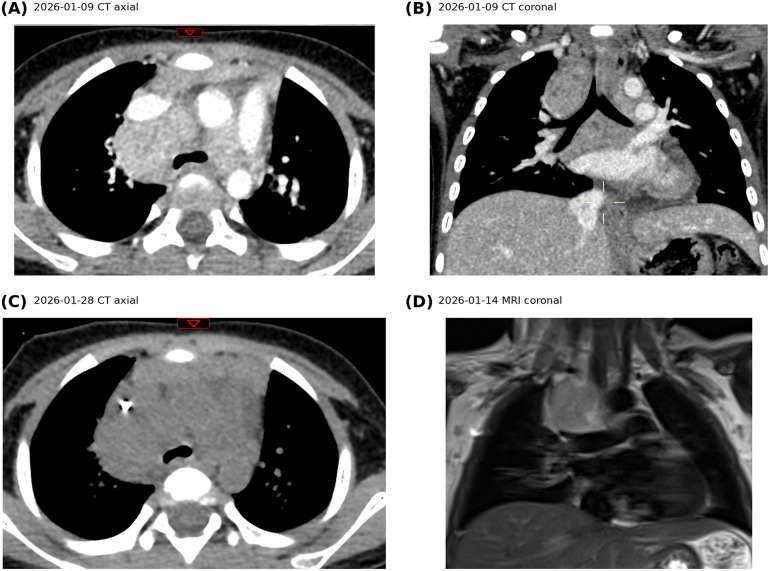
Representative imaging findings during hospitalization. (A) Axial contrast-enhanced chest CT on January 9, 2026 showing a mediastinal–hilar mass-like lesion with heterogeneous enhancement and airway compression. (B) Coronal contrast-enhanced CT on January 9, 2026 showing the relationship of the lesion to the airway, hila, and adjacent great vessels. (C) Axial non-contrast chest CT on January 28, 2026 showing persistent mediastinal–hilar disease without obvious reduction compared with January 9. (D) Coronal chest MRI on January 14, 2026 showing the mediastinal and hilar extent of disease. All images have been de-identified.
